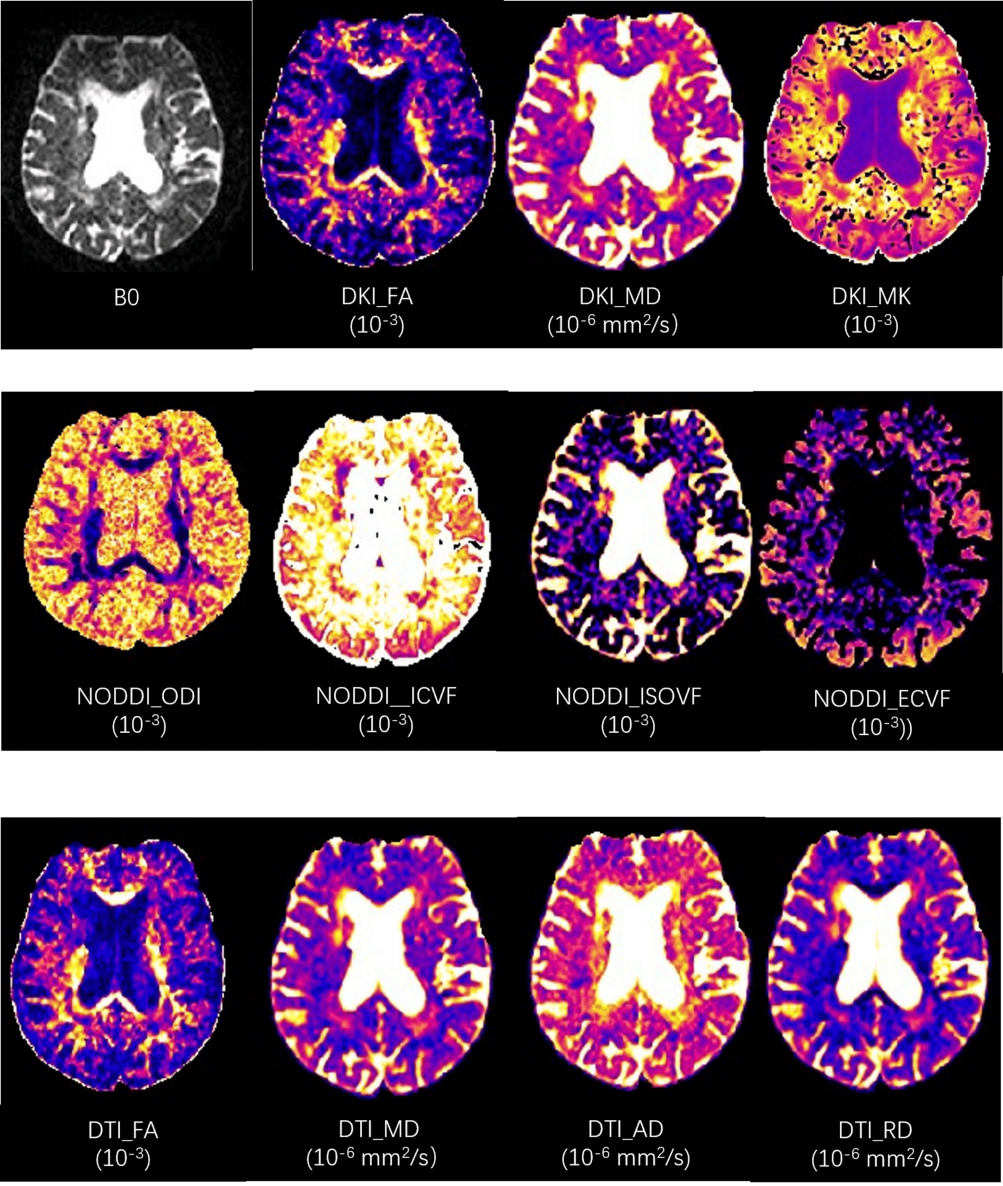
Images of diffusion parameters A 67-year-old male patient was treated in our hospital after a stroke. After two weeks of discharge, scale scores and MRI imaging were performed to evaluate post-infarction depression. The figure shows B0 and the parameters obtained by three diffusion models. The first behavior is the quantitative parameters of the diffusion kurtosis imaging (DKI) model, the second behavior is the quantitative parameters of the neurite orientation dispersion and density imaging (NODDI) model, and the third behavior is the quantitative parameters of the diffusion tensor imaging (DTI) model. The units of each parameter are in parentheses. DKI_FA: diffusion kurtosis imaging fractional anisotropy; DKI_MD: kurtosis imaging mean diffusivity; DKI_MK: Kurtosis imaging mean kurtosis; NODDI_ODI: neurite orientation dispersion and density imaging neurite orientation dispersion index; NODDI_ICVF: neurite orientation dispersion and density imaging intracellular volume fraction; NODDI_ECVF: neurite orientation dispersion and density imaging extracellular volume fraction; NODDI_ISOVF: neurite orientation dispersion and density imaging isotropic volume fraction; DTI_FA: diffusion tensor imaging fractional anisotropy; DTI_MD: diffusion tensor imaging mean diffusivity; DTI_AD: diffusion tensor imaging axial diffusivity; DTI_RD: diffusion tensor imaging radial diffusivity
